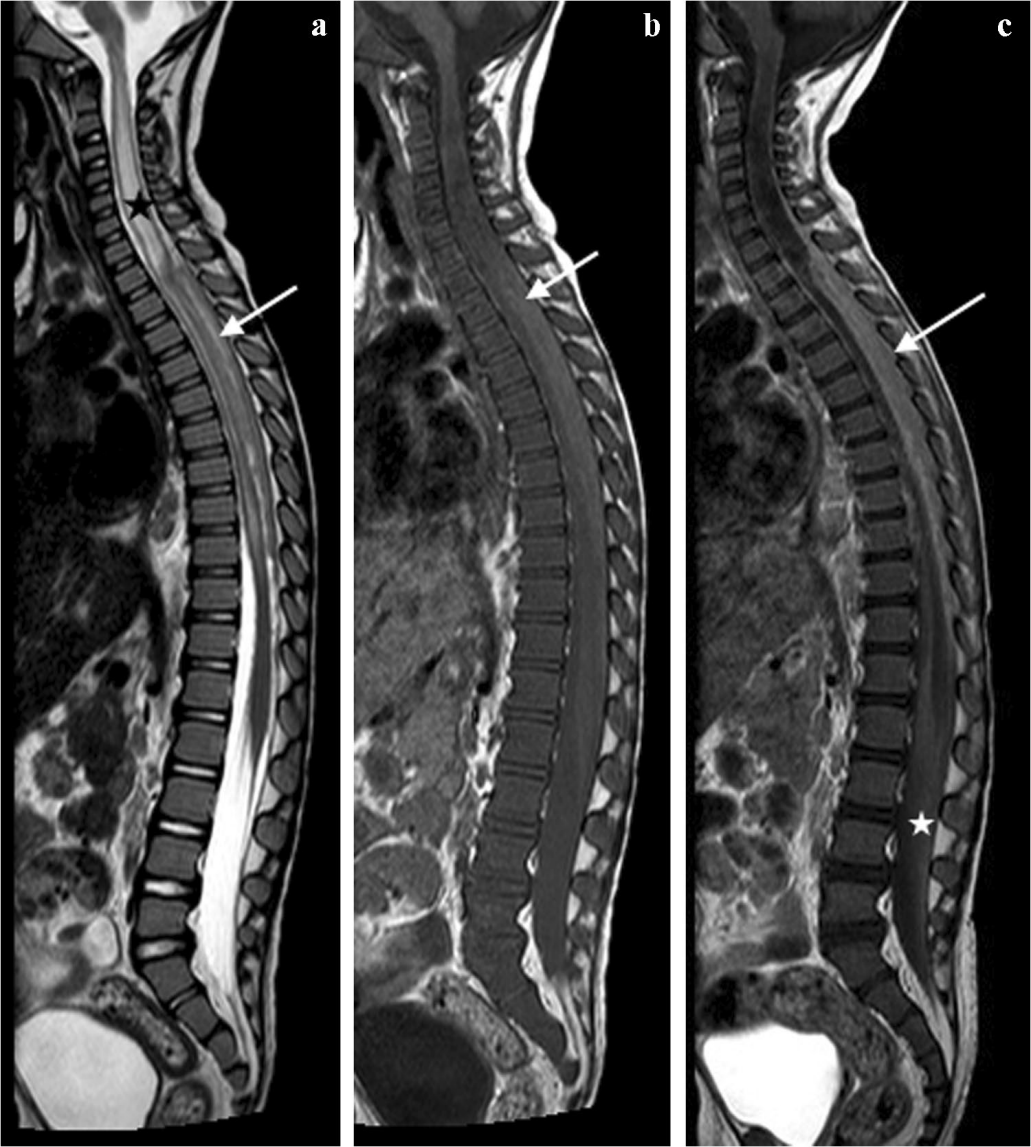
Sagittal magnetic resonance images of the spine in a 5-year-and-3-month-old girl with tuberculous meningitis and progressive lower limb weakness. a T2 image shows a hypointense posterior epidural mass extending from T2 to T9 (*arrow*) and compressing the cord anteriorly. There is cord oedema proximal to the mass (*asterisk*). b, c  T1 pre- (b) and post- (c) gadolinium show the mass to be homogeneously enhancing (*arrows*). There is also nerve root enhancement (*asterisk in *c)
